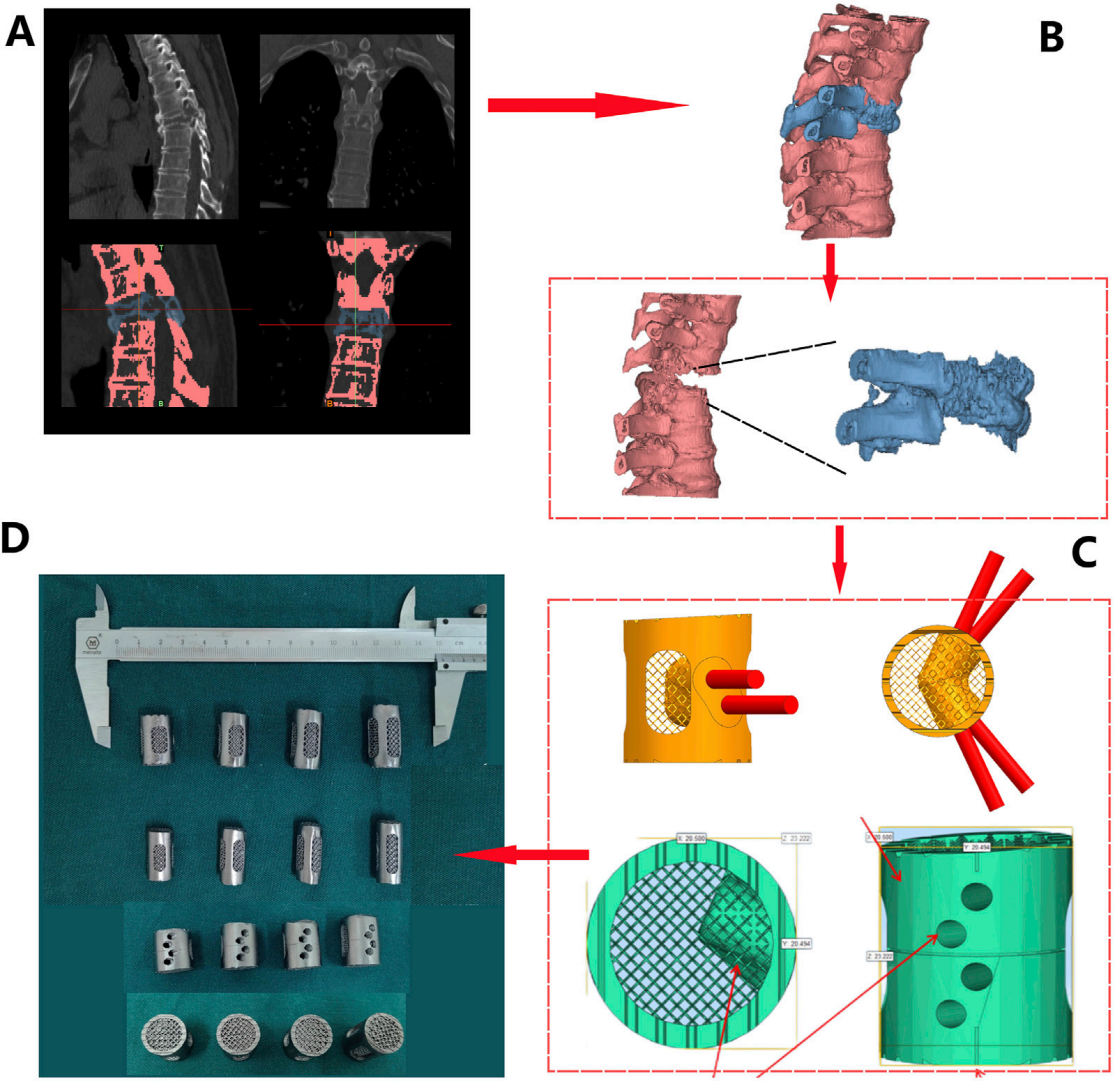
Prosthesis design and fabrication process for patient 2: (A) CT Scan Images: obvious bone destruction in the T6 vertebral body, with compression and deformation of the vertebral body (1/2). T6 and adjacent vertebrae were differentiated by threshold adjustment (3/4); (B) Three-dimensional reconstruction based on CT data (red - normal vertebrae, blue - T6); (C) Prosthesis design was conducted based on measurement, including a 3° retroversion angle (1), 30° and 45° screw holes (2), polished lateral surface design (3), and roughened superior and inferior surfaces (4); (D) Four sizes of porous tantalum prostheses with varying heights (21/23/25/27 mm) were fabricated via 3D printing.
